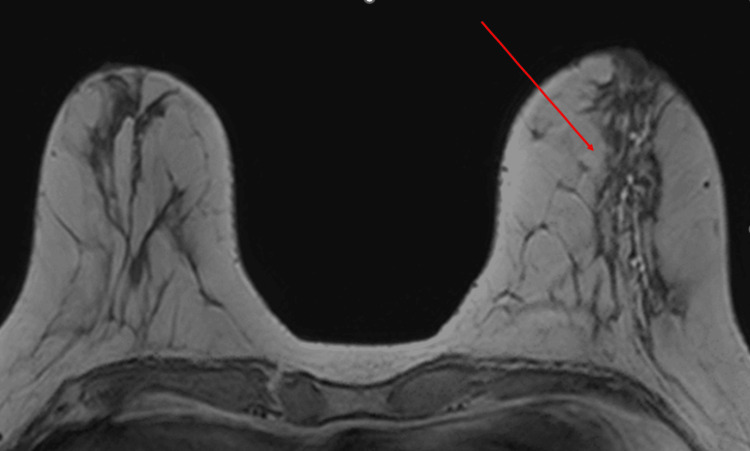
Breast MRI axial T1 pre-contrast: T1-hyperintense ductal ectasia in the central left breast (indicated by arrow).
